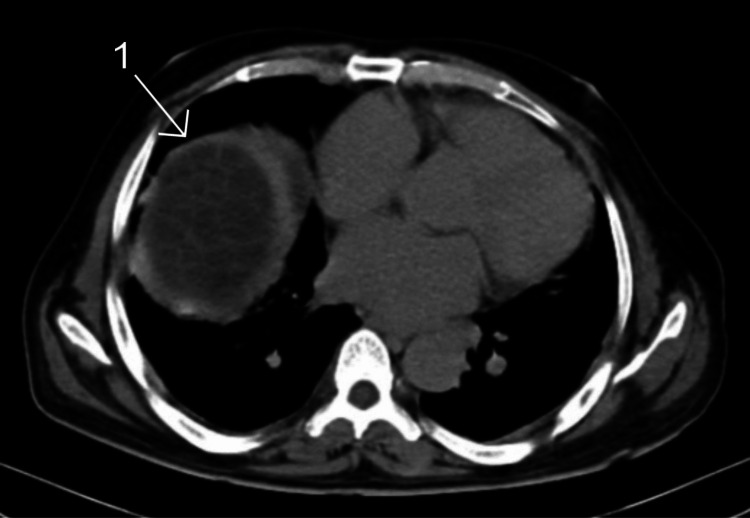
Computed tomography report showing a hydatid cyst in the lung. 1: A hydatid cyst in the right lung containing multiple daughter cysts.
